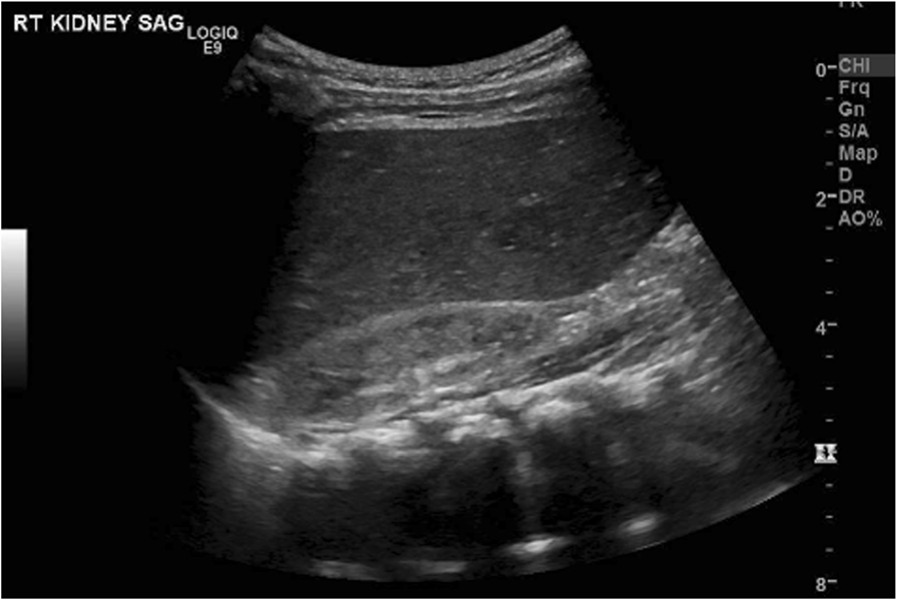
Renal Ultrasound of Left Kidney - 3.9 × 2.3 × 2.7 cm
